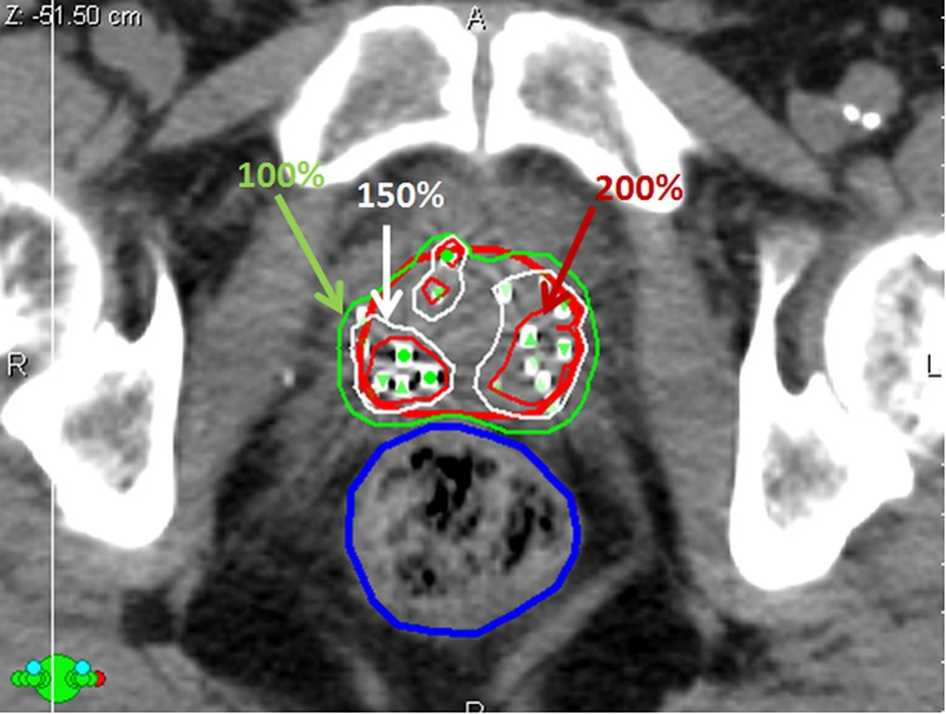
Post-implant dosimetric quality assurance (QA) for a typical LDR BT treatment plan 1 month following implantation. Imaging the prostate (*red*) and rectum (*blue*) interface can be challenging on CT-guided post-implant QA. Isodose lines are shown as a percentage of the prescription dose (145 Gy). Sources are identified as *green circles* with *upward-directed* and *downward-directed triangles* representing out-of-plan sources. Treatment planning system is VariSeed 8.0 (Varian Inc. Palo Alto, CA, USA)
